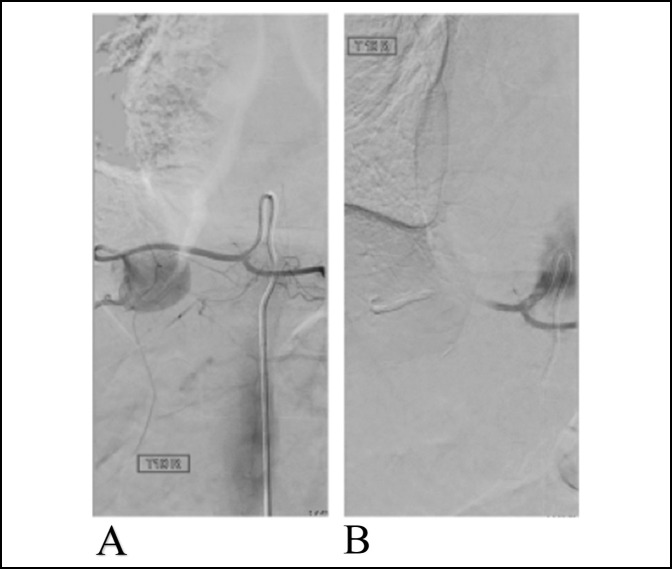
Radiograph of the arterial angiogram of the embolization procedure. A, Photograph showing filling of the pseudoaneurysm with contrast. B, Postembolization with ONYX 18 with no flow into the pseudoaneurysm.
